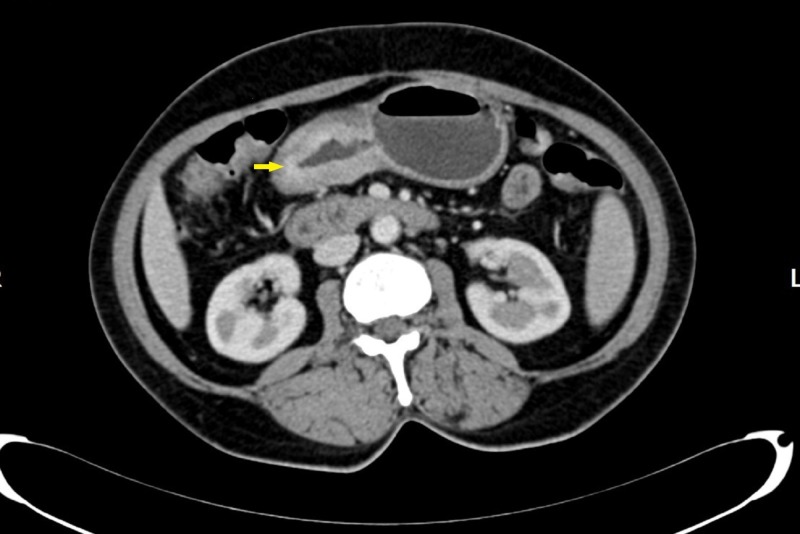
CECT showing enhancing wall thickening of the antropyloric region of the stomach (arrow) CECT: contrast-enhanced computed tomography
